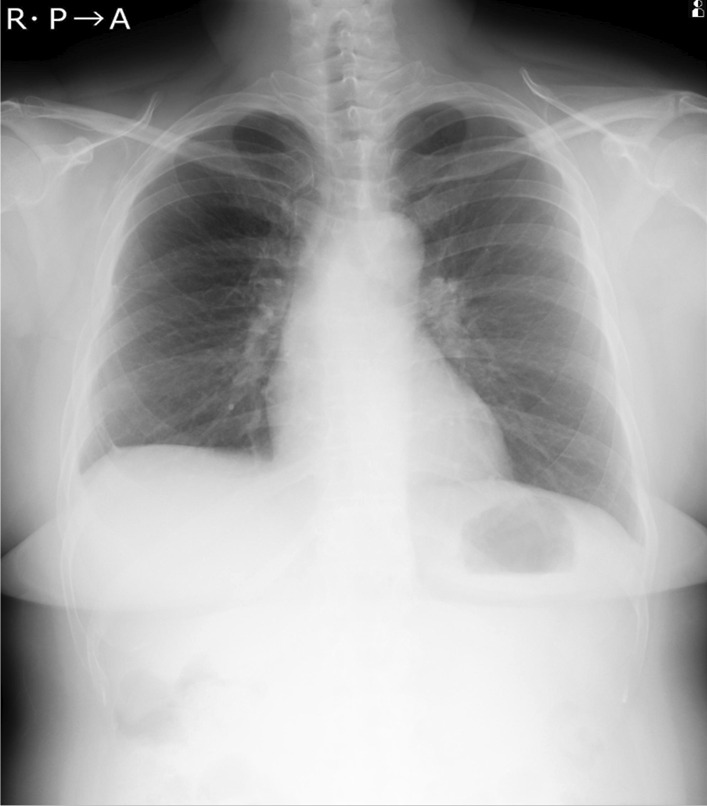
Chest X-ray at 6 months after administration of steroid showing a reduction in the upper mediastinal widening
